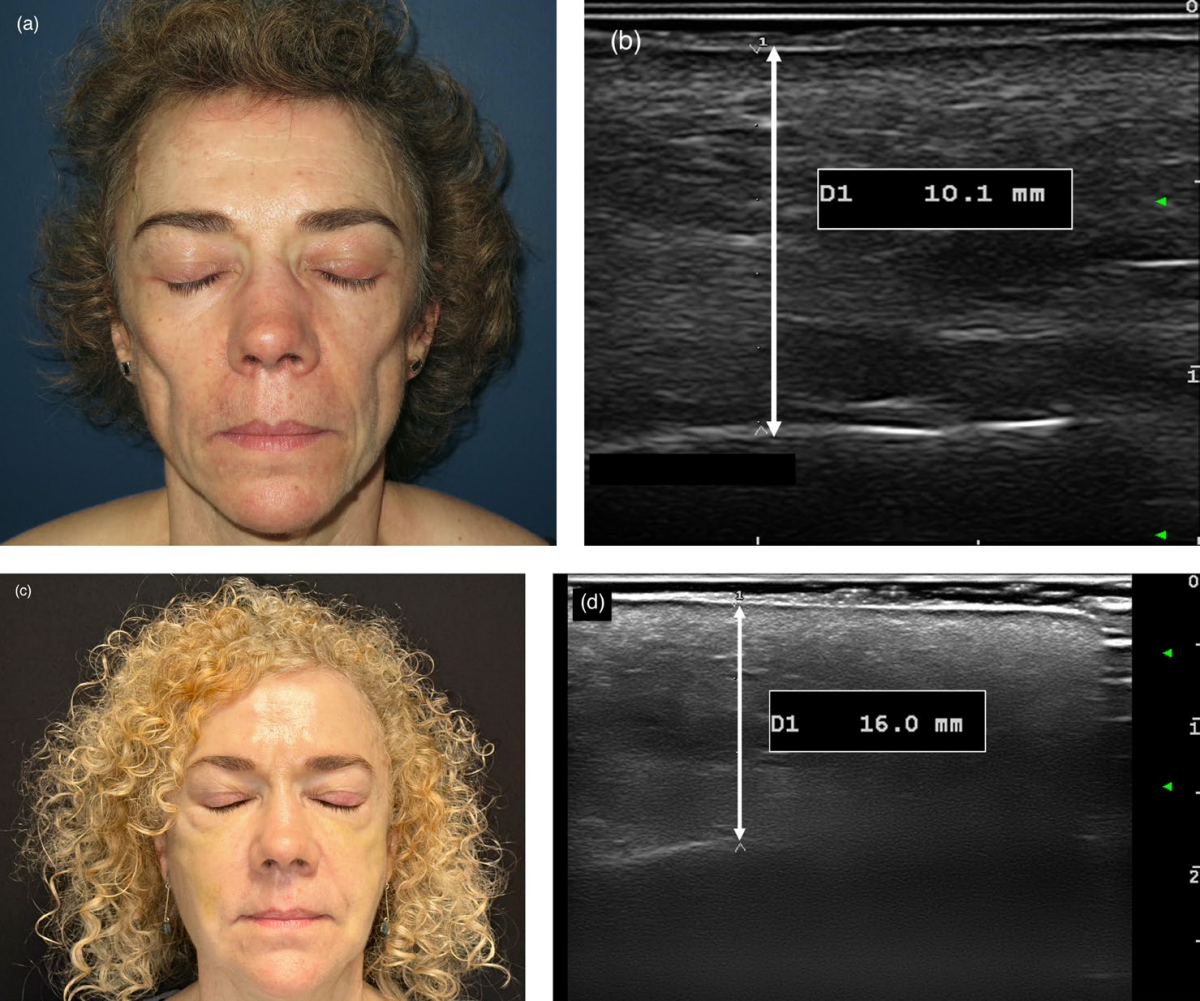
“58‐year‐old female with marked bilateral malar and cheek atrophy due to dermatomyositis.” (a) “Clinical image before lipofilling.” (b) “High‐frequency ultrasound (HFU) measure of skin thickness from the epidermis to the underlying bone in the malar area before lipofilling.” (c) “Clinical image 2 weeks after the 3rd lipofilling session.” (d) “HFU measure of skin thickness in the malar area 2 weeks after the 3rd lipofilling session.”
